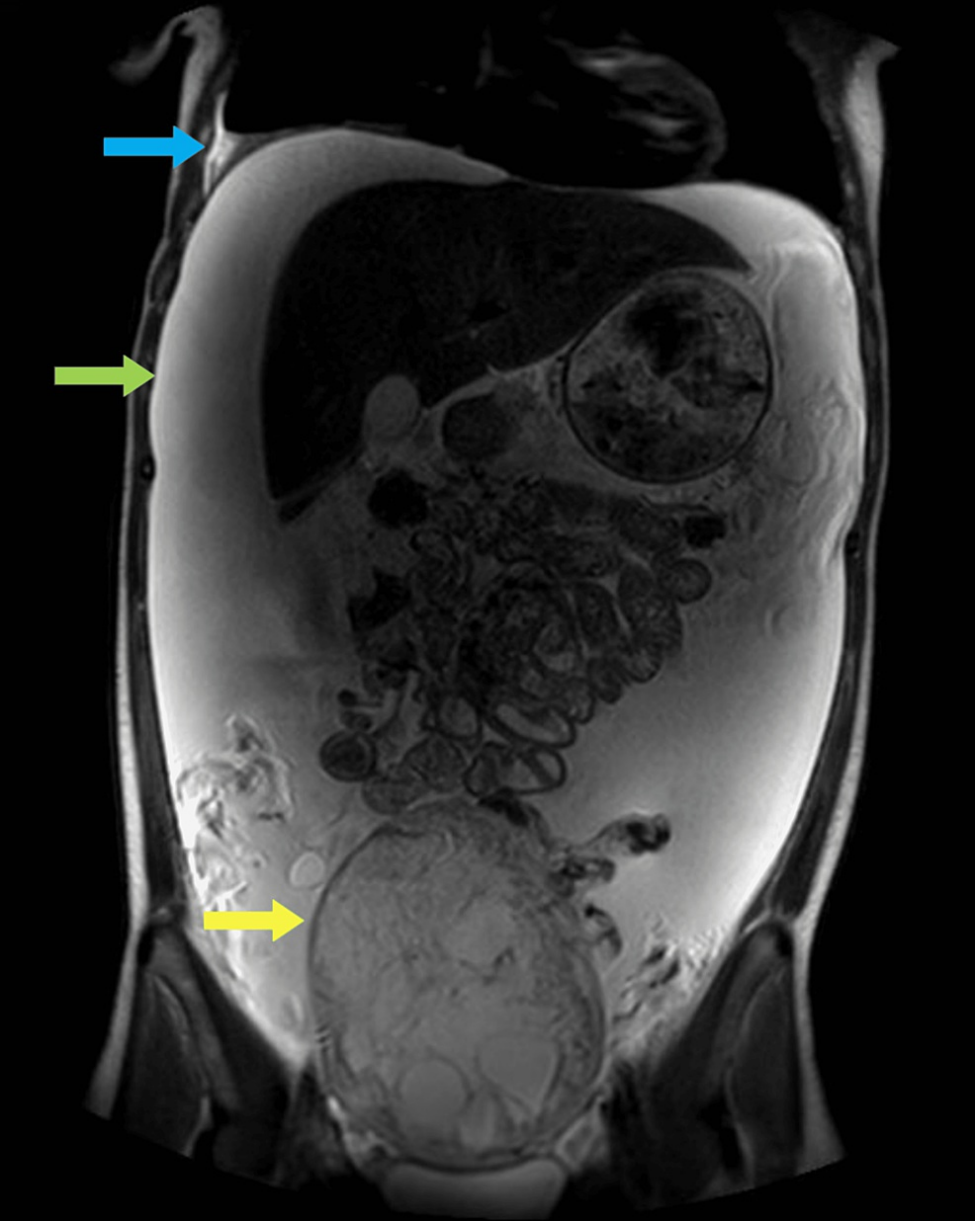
Magnetic resonance imaging with gadolinium. A large 14.6 × 11.8 × 8.4 cm peripherally enhancing complex cystic mass appeared to be arising from the left adnexa and showed internal septations which seemed to be enhancing as well (yellow arrow). The mass was abutting the dome of the bladder with no definite invasion. Secondary large-volume ascites (green arrow) and a moderate right pleural effusion with underlying atelectasis (blue arrow) were noted.
